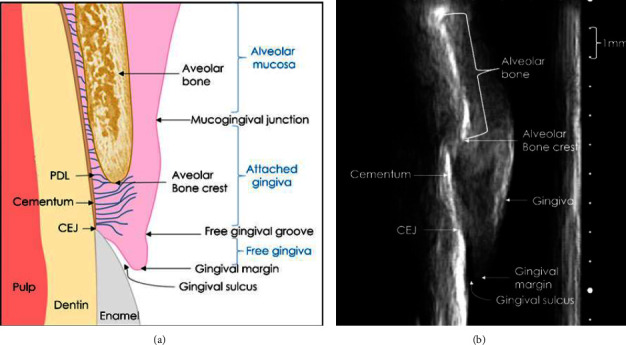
The anatomy of the periodontium compared to an ultrasound image of tooth 11. The (a) was taken from Chan et al. [37] with modification.
